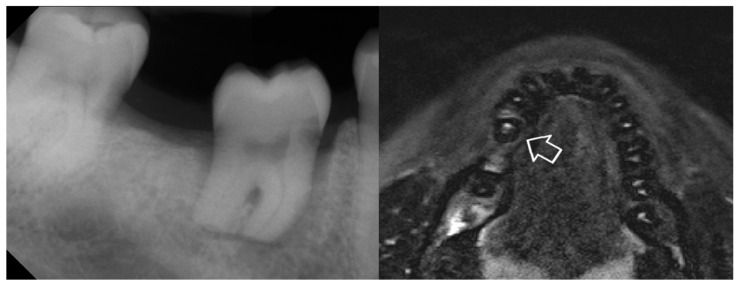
Radiographic control 4 weeks postoperatively. (Left), intraoral radiograph with still-missing signs of PDL regeneration or reperfusion. (Right), contrast-enhanced MRI with uptake of the contrast medium in the pulp chamber (arrow) already confirming reperfusion.
